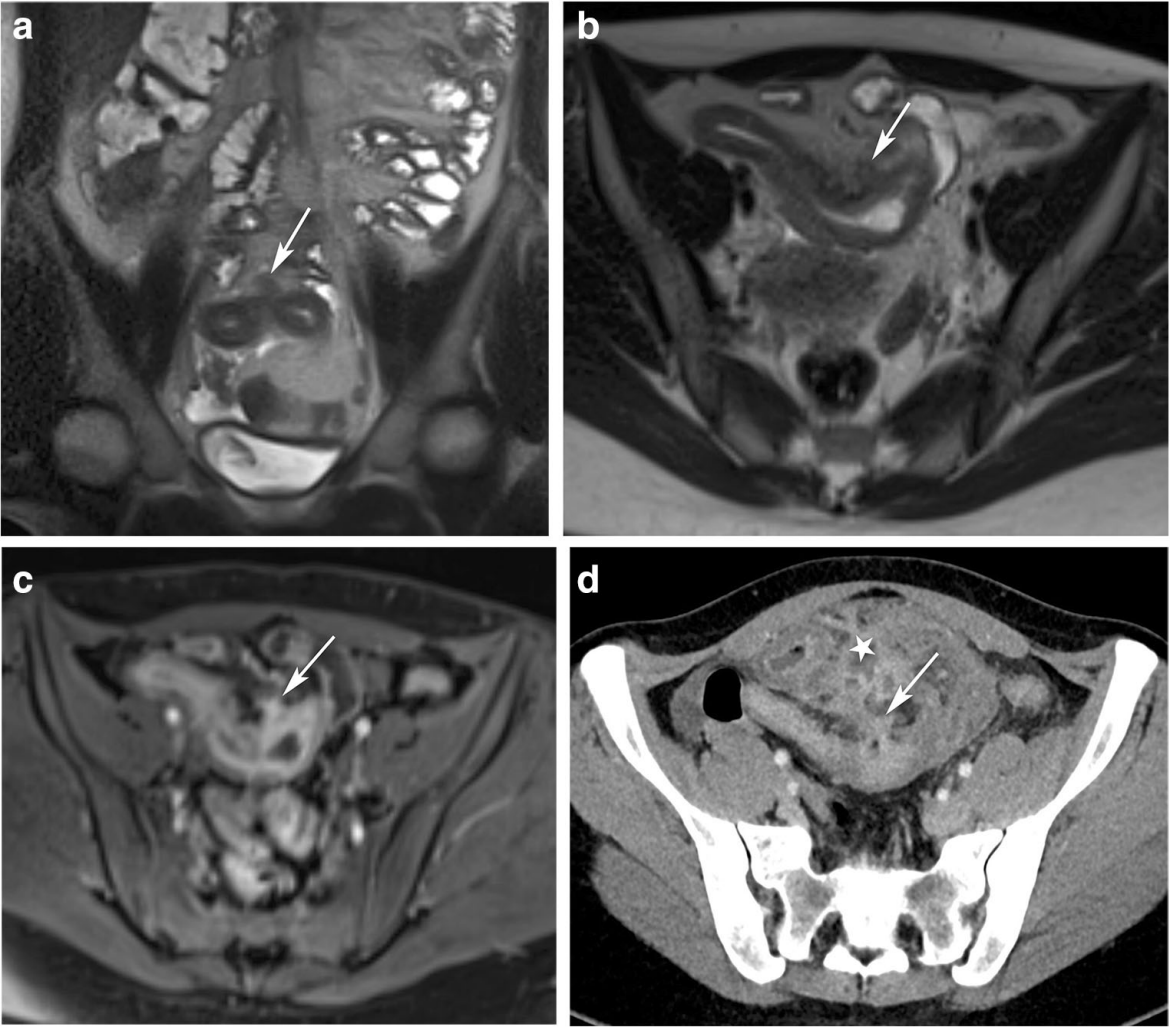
A 26-year-old woman with Crohn’s disease and diarrhea: follow-up of a sinus tract. Coronal (a) and axial (b) T2-weighted MR images show a single sinus tract within the preterminal ileum (arrow), with marked enhancement of the bowel wall (arrow) on post-contrast imaging (c) and a location within a stenotic segment. Eleven months later, the patient presented with an acute abdomen. Axial contrast-enhanced CT image (d) shows a large abscess formation (star) originating from the bowel segment that contained the sinus tract (arrow)
